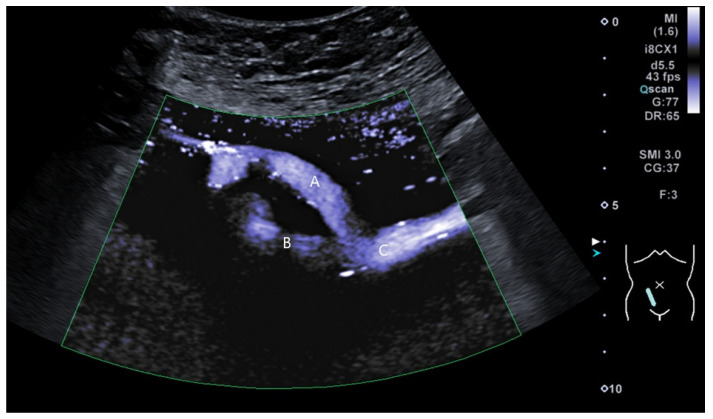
The allograft renal vein is shown anastomosed to the distal part of the external iliac vein. A—allograft renal vein; B—proximal external iliac vein; C—distal external iliac vein. Ultrasound superb vascular imaging mode (SMI) mode.
